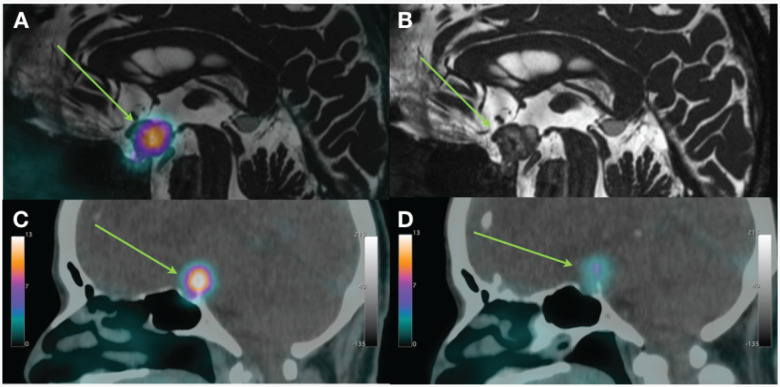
(A) Fusion imaging of gallium-68-DOTATOC positron emission tomography –computed tomography (^68^Ga-DOTATOC PET-CT) and brain magnetic resonance imaging showing high uptake by the patient's suprasellar hemangioblastoma, sagittal view; (B) brain magnetic resonance imaging of the suprasellar hemangioblastoma without fusion imaging, sagittal view. ^68^Ga-DOTATOC PET-CT imaging of the suprasellar hemangioblastoma (C) before lanreotide treatment, sagittal view, and (D) after lanreotide treatment, sagittal view.
